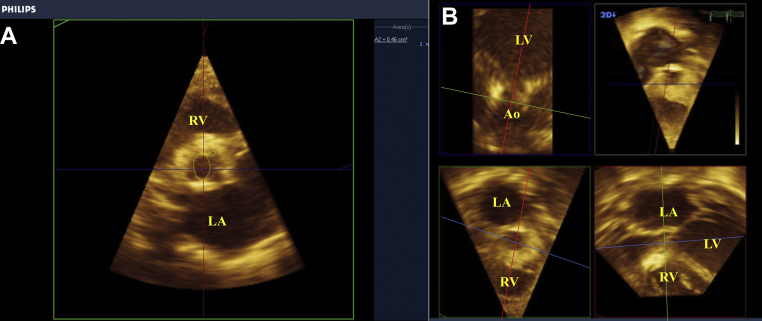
Three-dimensional TTE from the left parasternal window shows the aortic valve short-axis view at the midsystolic phase. (A) The 3D-TTE data set was obtained by using 3D analysis software (*top right*). The data set was cropped to create a 2-chamber (*top left*) and a long-axis view (*bottom right*). These made the cross-sectional view at the level of the edges of the aortic valve (*bottom left*). (B) The traced anatomical AVA 3D-TTE planimetry in the zoomed cross-sectional view at the level of the edges of the aortic valve was 0.46 cm^2^ (1.39 cm^2^/m^2^). *Ao*, Aorta; *LA*, left atrium; *LV*, left ventricle; *RA*, right atrium; *RV*, right ventricle.
